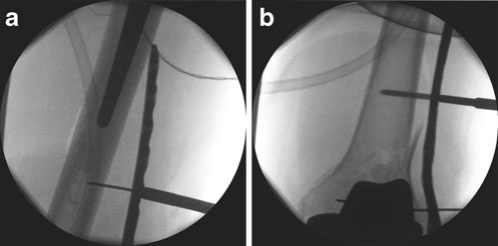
Intraoperative X-ray pictures demonstrating the minimally invasive technique of closed reduction with temporary proximal plate fixation (a) and reduction of the fragments by the screws through the plate (b)
